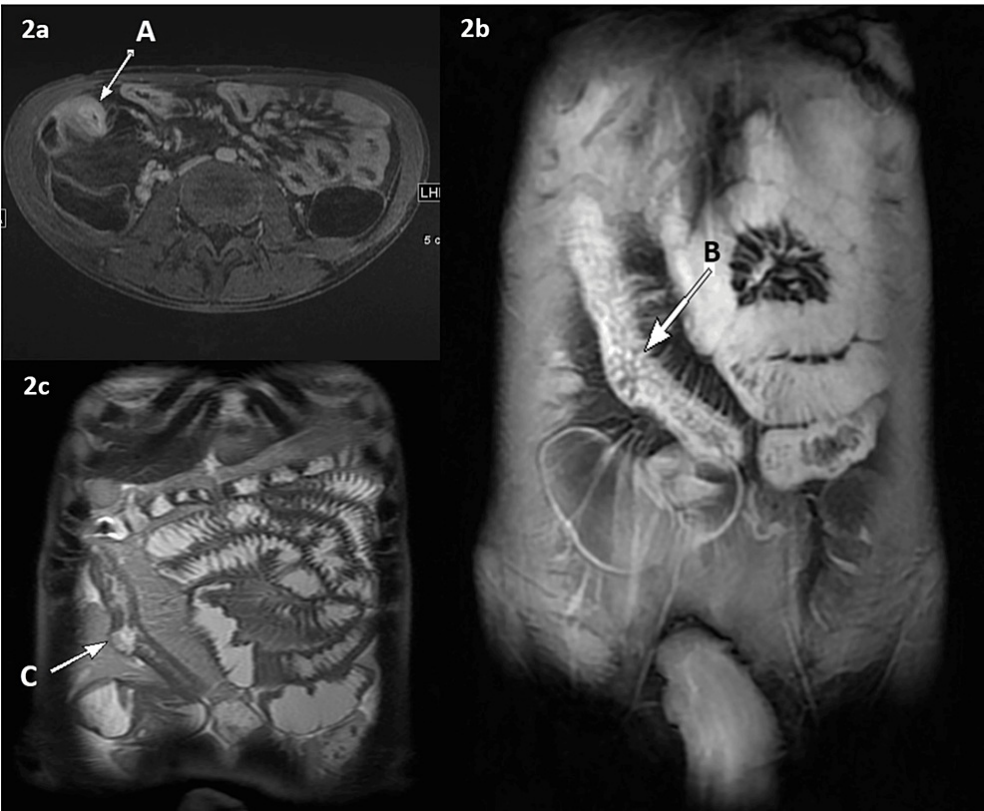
MRI image analysis Axial (2a) and coronal (2b) T1 fat-suppressed post-contrast images of the abdomen and pelvis and coronal (2c) T2 with oral volume demonstrate long segment of circumferential wall thickening and mucosal enhancement involving the distal ileum A: an entero-enteric fistula seen in the right iliac fossa; B: mucosal enhancement and congested mesenteric vessels; C: at least one deep mucosal ulceration seen in the distal ileum
